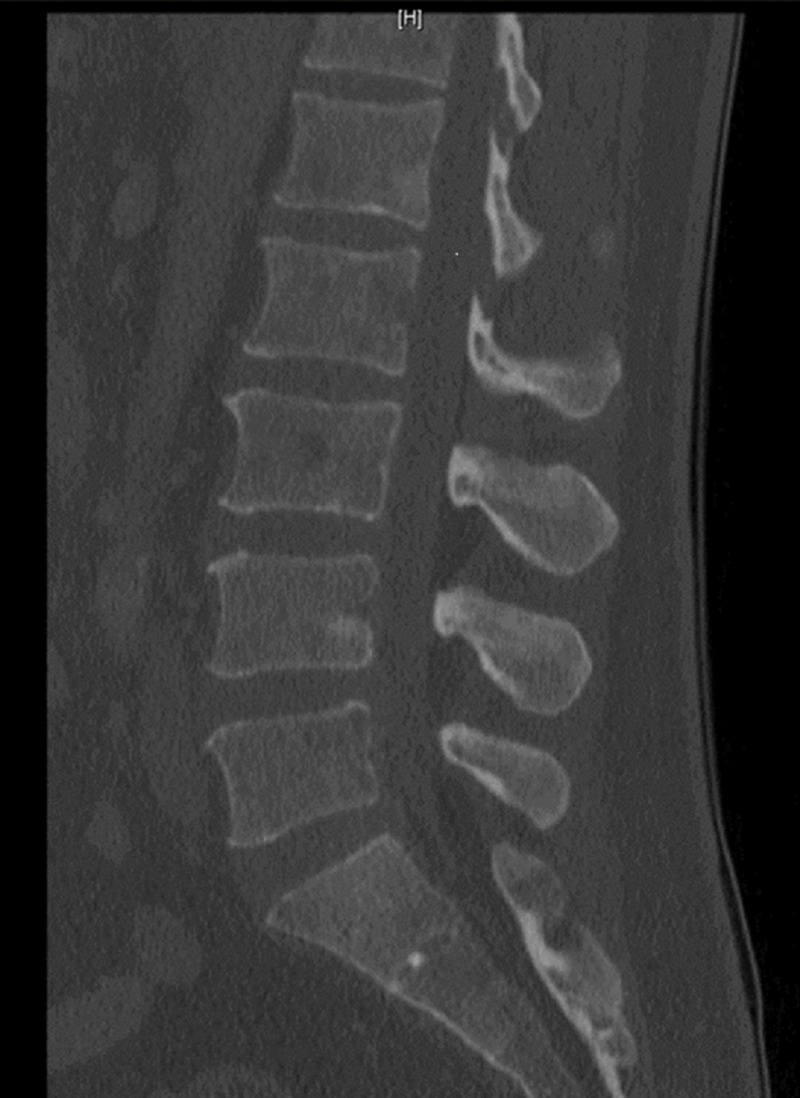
Sagittal view of CT lumbar spine revealing multiple small, nonspecific, round, radiolucent, and sclerotic bone lesions
